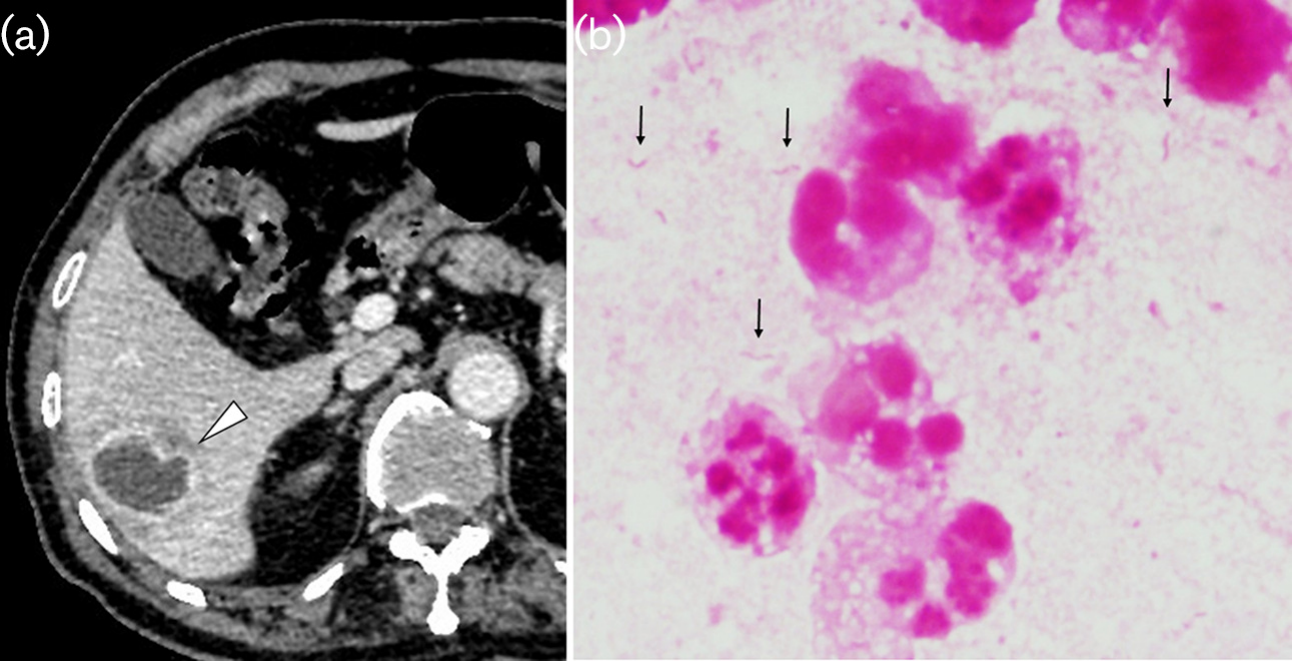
Contrast-enhanced computed tomography showing the liver abscess (a) and Gram-negative spiral bacilli in the pus (b).
